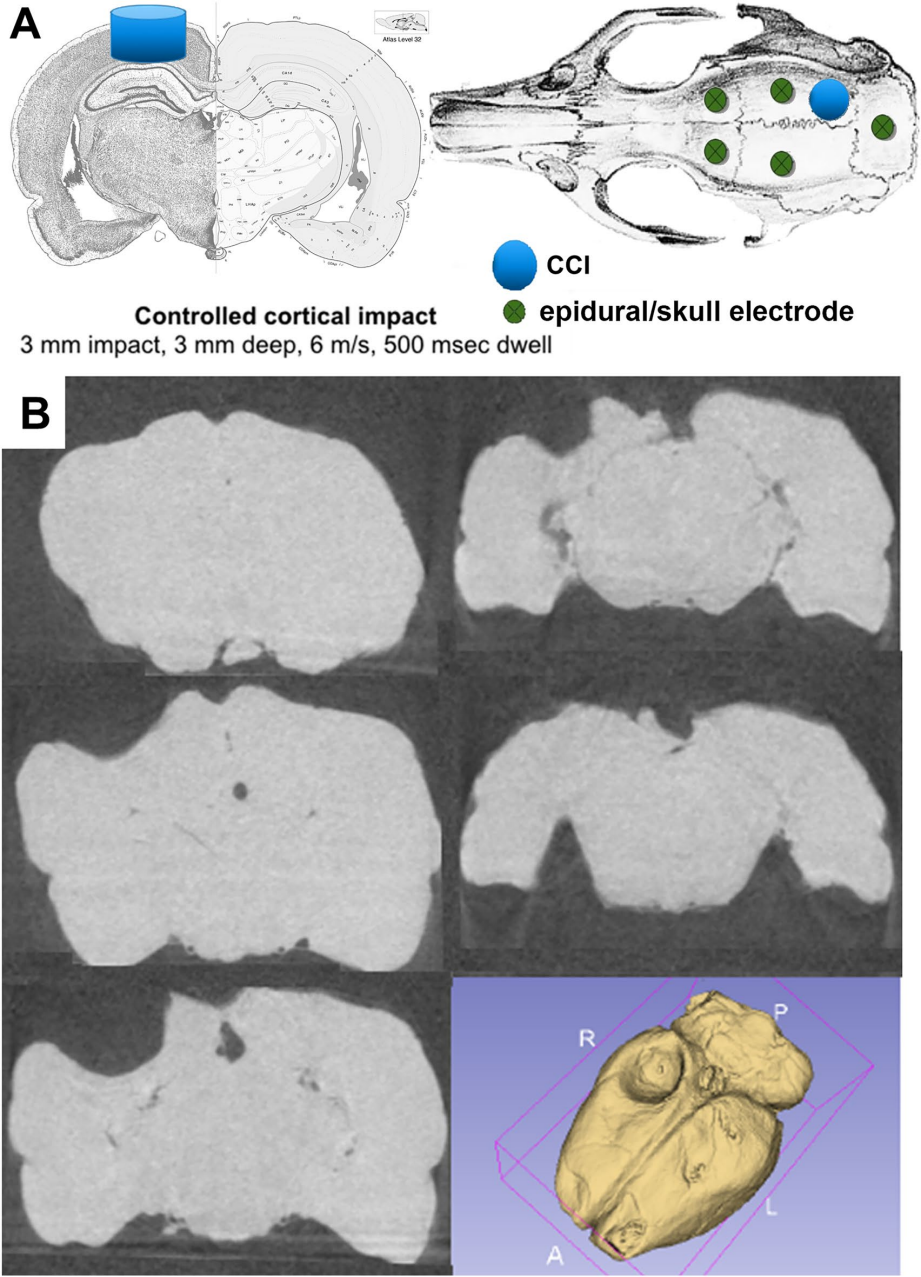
Traumatic brain injury. (A) A controlled cortical impact (CCI) is targeted to the posterior cortex, with a depth (3mm) chosen to abut the hippocampus. Epidural electrodes are placed bilaterally anterior and posterior, with a reference electrode in the posterior skull. (B) CT imaging with coronal sections and reconstruction demonstrating a representative lesion at 6 months following CCI.
